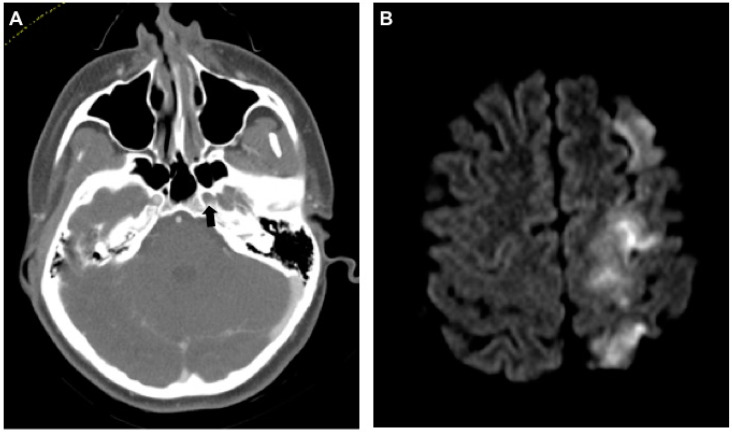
Dissection of the left internal carotid artery (ICA) with severe neurologic sequelae. (A) Axial computed tomography angiogram (CT-A) of the skull base shows no contrast agent in the ICA within the foramen carotideum on the left (black arrow). (B) Same-day magnetic resonance imaging (MRI) (diffusion weighted imaging (DWI), b800) shows ipsilateral infarction of the anterior and middle cerebral artery territories.
